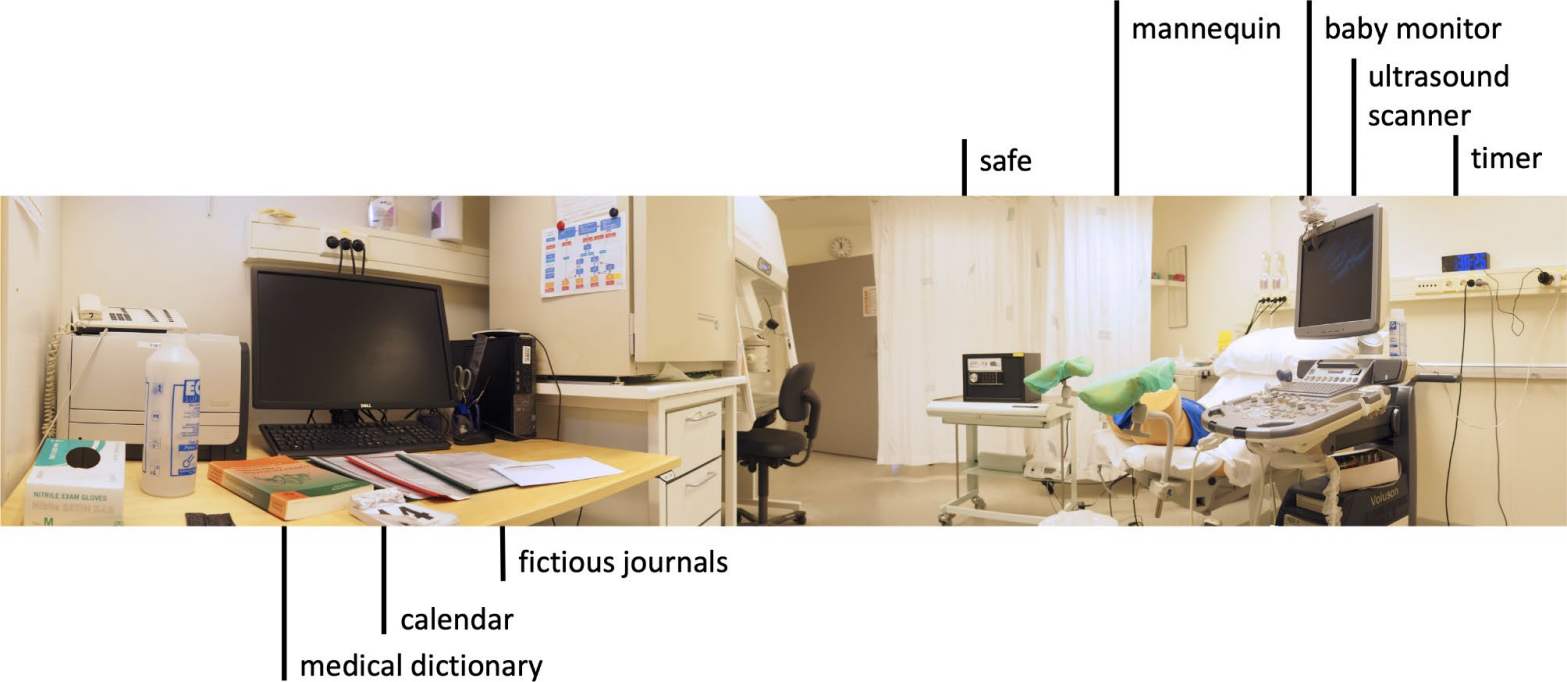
Arrangement of game items in the outpatient room. Before starting the escape room game, the gamemaster arranges all props and clinical items in the room (left to right): the medical dictionary, tear-off calendar showing the game’s date and fictious journals on the desk; document safe with the gestational wheel and address book locked inside; mannequin on the examination chair; ultrasound scanner; baby monitor to view the examination area; large-display countdown timer set to 30 min
